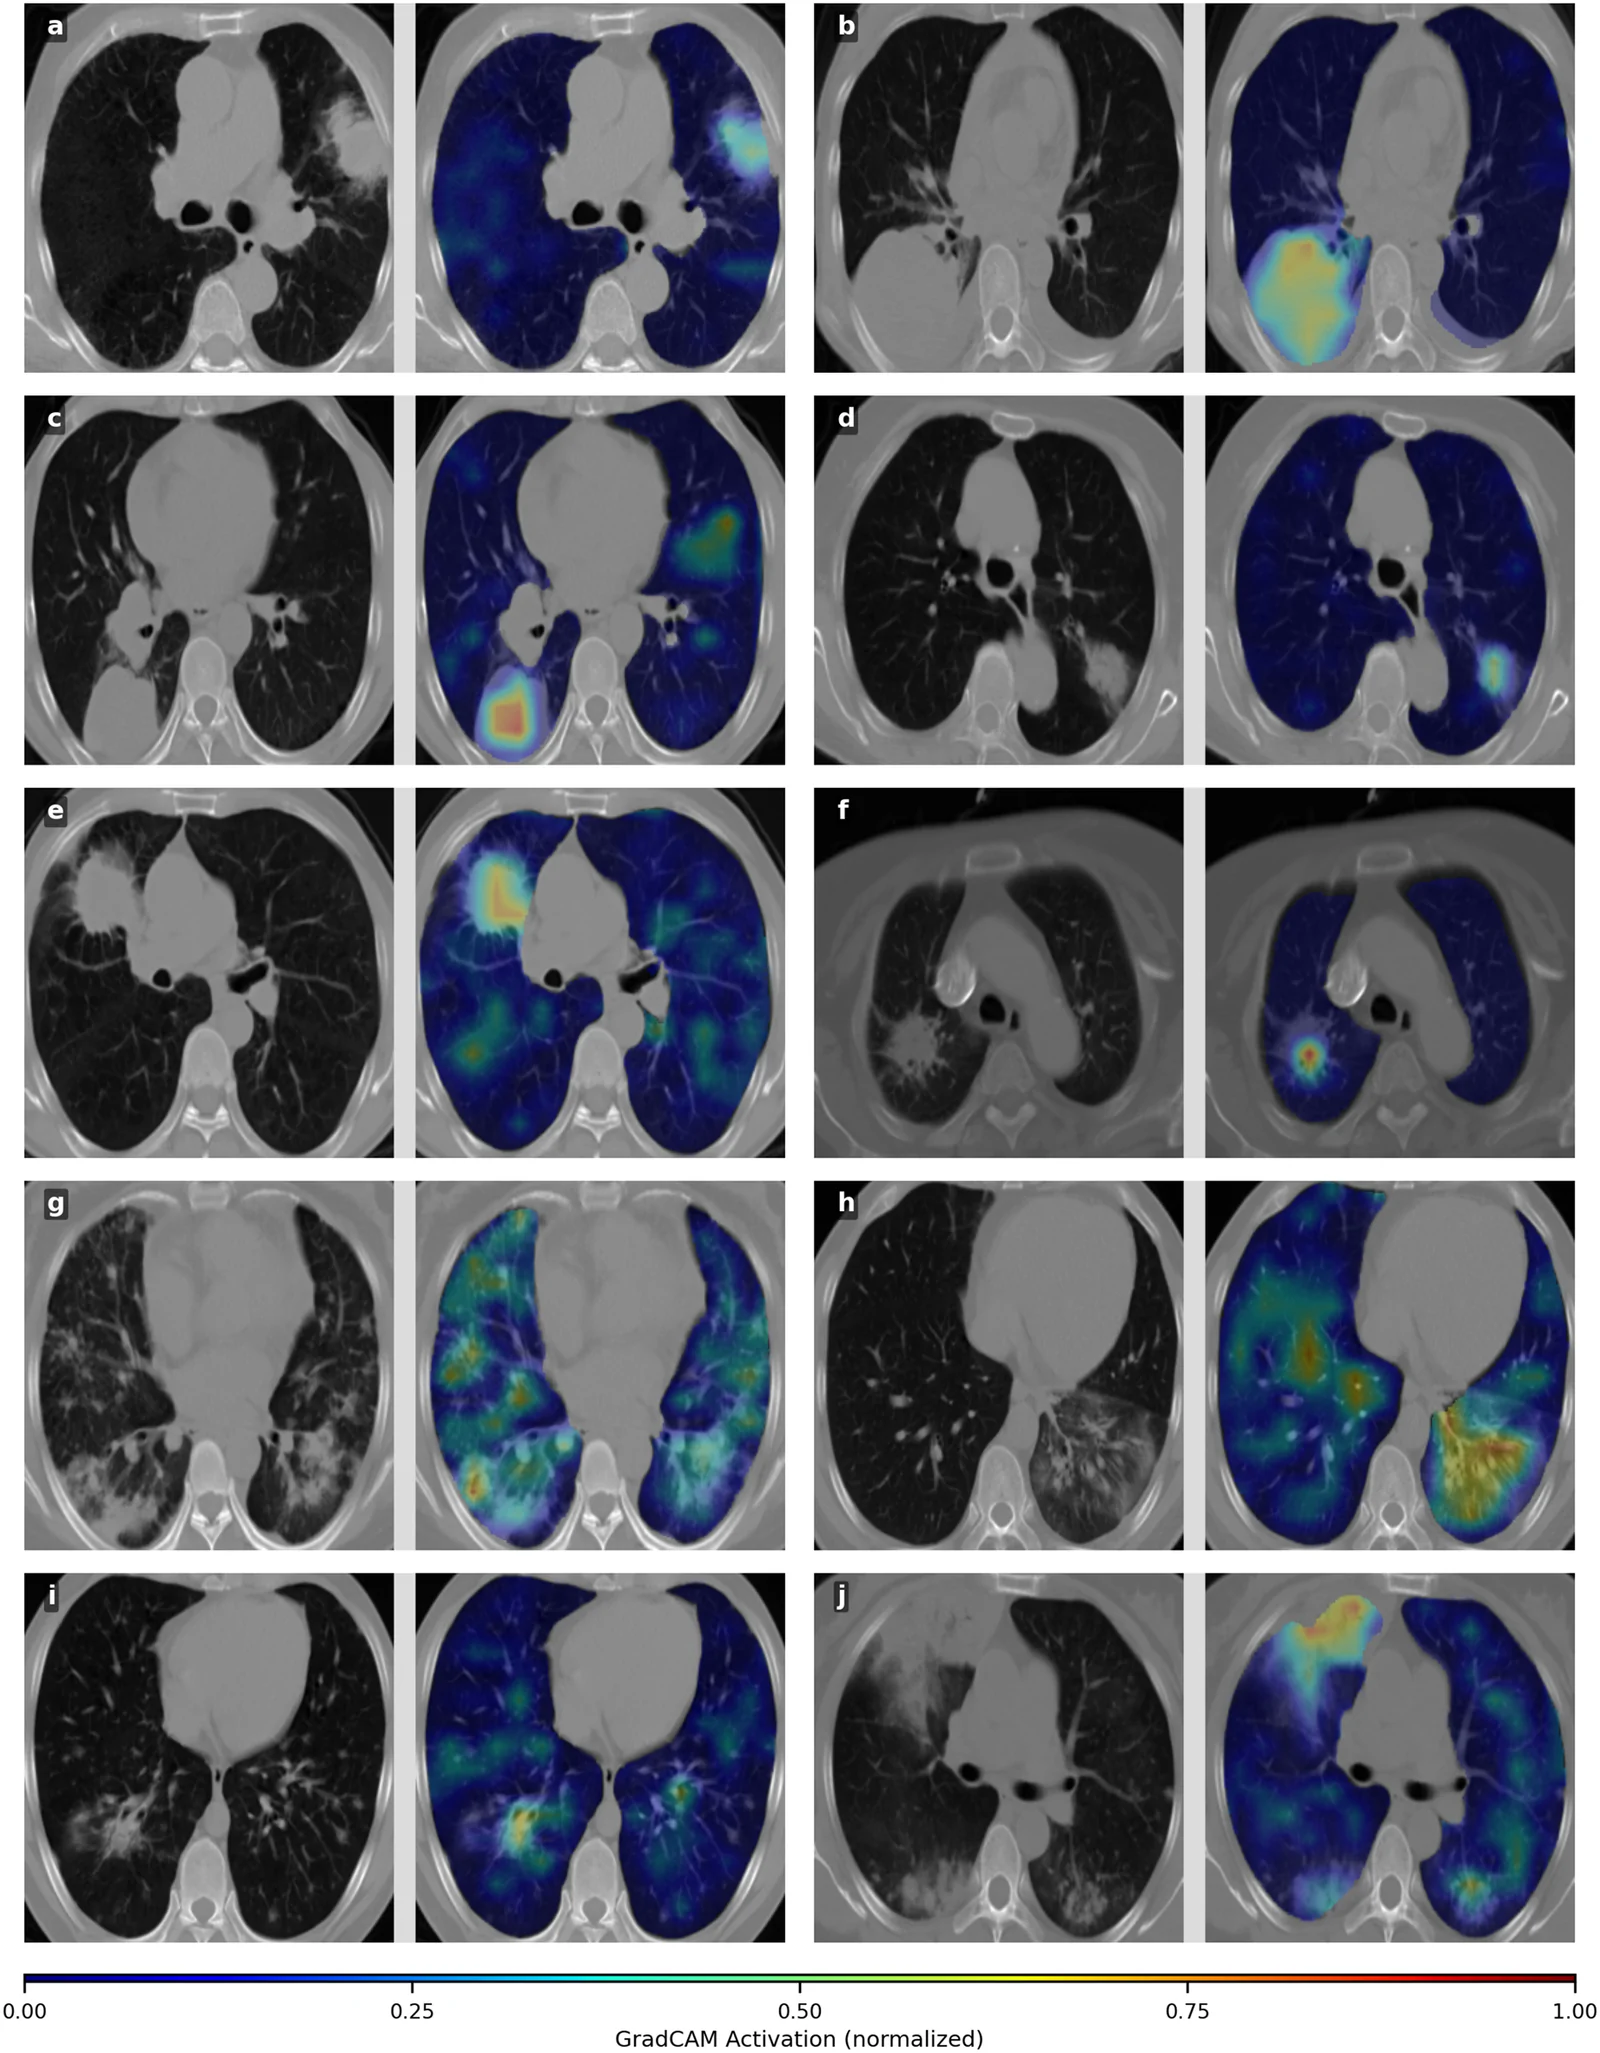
Gradient-weighted class activation mapping visualizations of representative prognosis predictions. For each representative case a–j, the original axial computed tomography image is shown on the left and the corresponding gradient-weighted class activation mapping overlay is shown on the right. The activation maps are normalized to the range of 0–1, with warmer colors indicating image regions that contributed more strongly to the model prediction
